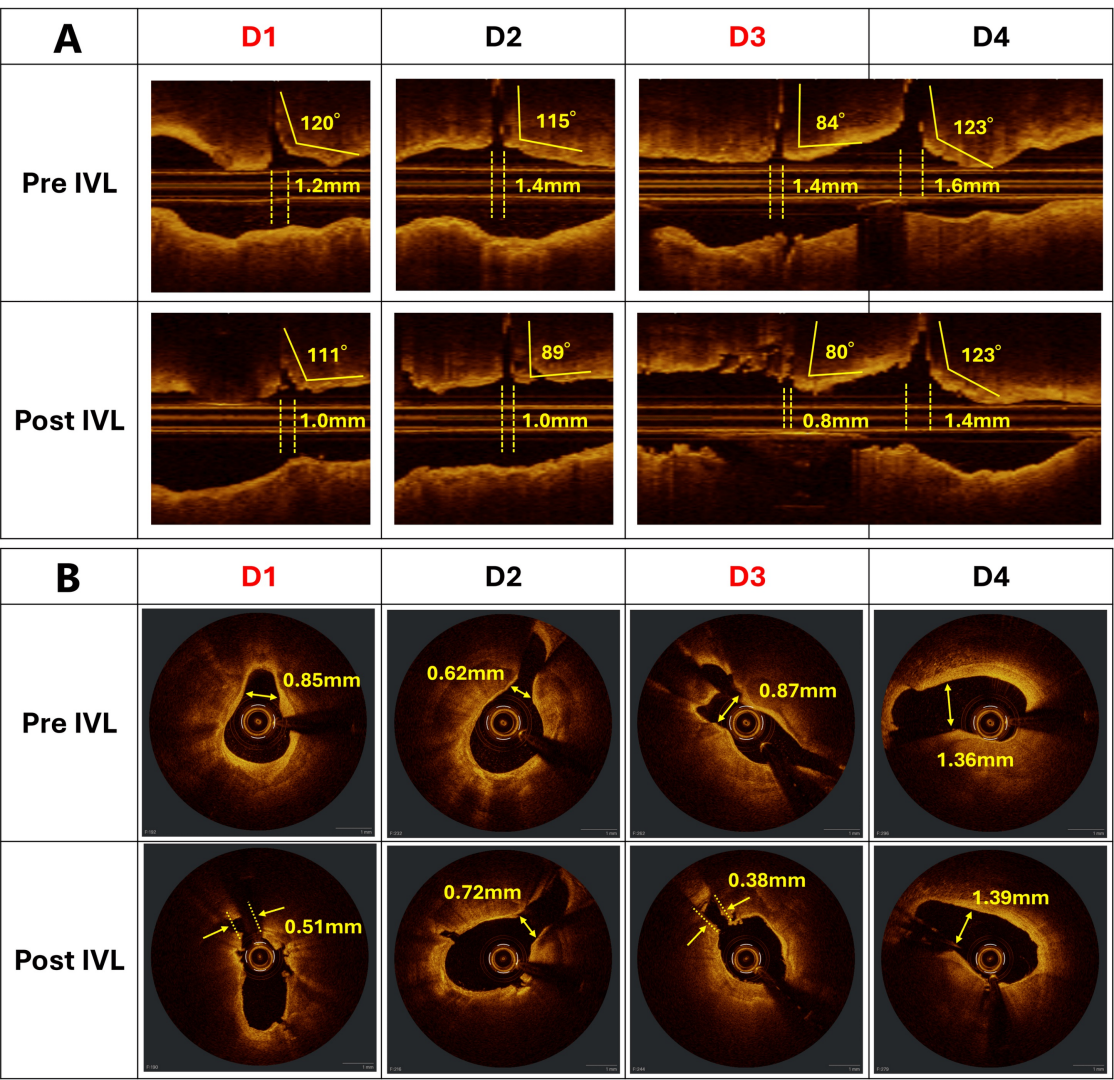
OCT findings before and after IVL A) On longitudinal OCT images before and after IVL, solid lines indicate the CT angles of side branches (D1–D4), and dashed lines indicate BP-CT lengths. Numerical values are provided in the main text. B) On cross-sectional images, D1 and D3 showed narrowing at the entry point due to fractured calcifications post-IVL. Yellow arrows indicate the width of the side branches before and after IVL (D1: 0.85 → 0.51 mm, D2: 0.62 → 0.72 mm, D3: 0.87 → 0.38 mm, D4: 1.36 → 1.39 mm), highlighting significant narrowing in D1 and D3. IVL: intravascular lithotripsy, OCT: optical coherence tomography
